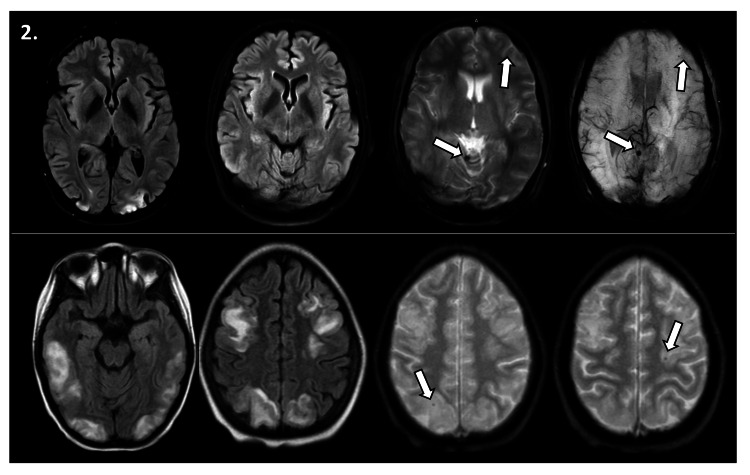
Microhemorrhages in the context of reversible posterior leukoencephalopathy syndrome Two different patients, one in the upper panel and another in the lower panel. (Upper panel, center, and left images) Axial brain magnetic resonance imaging (MRI) in FLAIR sequence, showing bioccipital hyperintense areas consistent with vasogenic edema. (Upper panel, center, and right images) GRE and SWI sequences, respectively, demonstrating small hypointense areas indicative of microhemorrhages. These microhemorrhages are observed in the infratentorial region of the cerebellar vermis and in the supratentorial left frontal lobe. (Lower panel, center, and left images) Extensive bilateral frontal, occipital, and temporal vasogenic edema areas. (Lower panel, center, and right images) Isolated microhemorrhages in the same regions as the described vasogenic edema.
